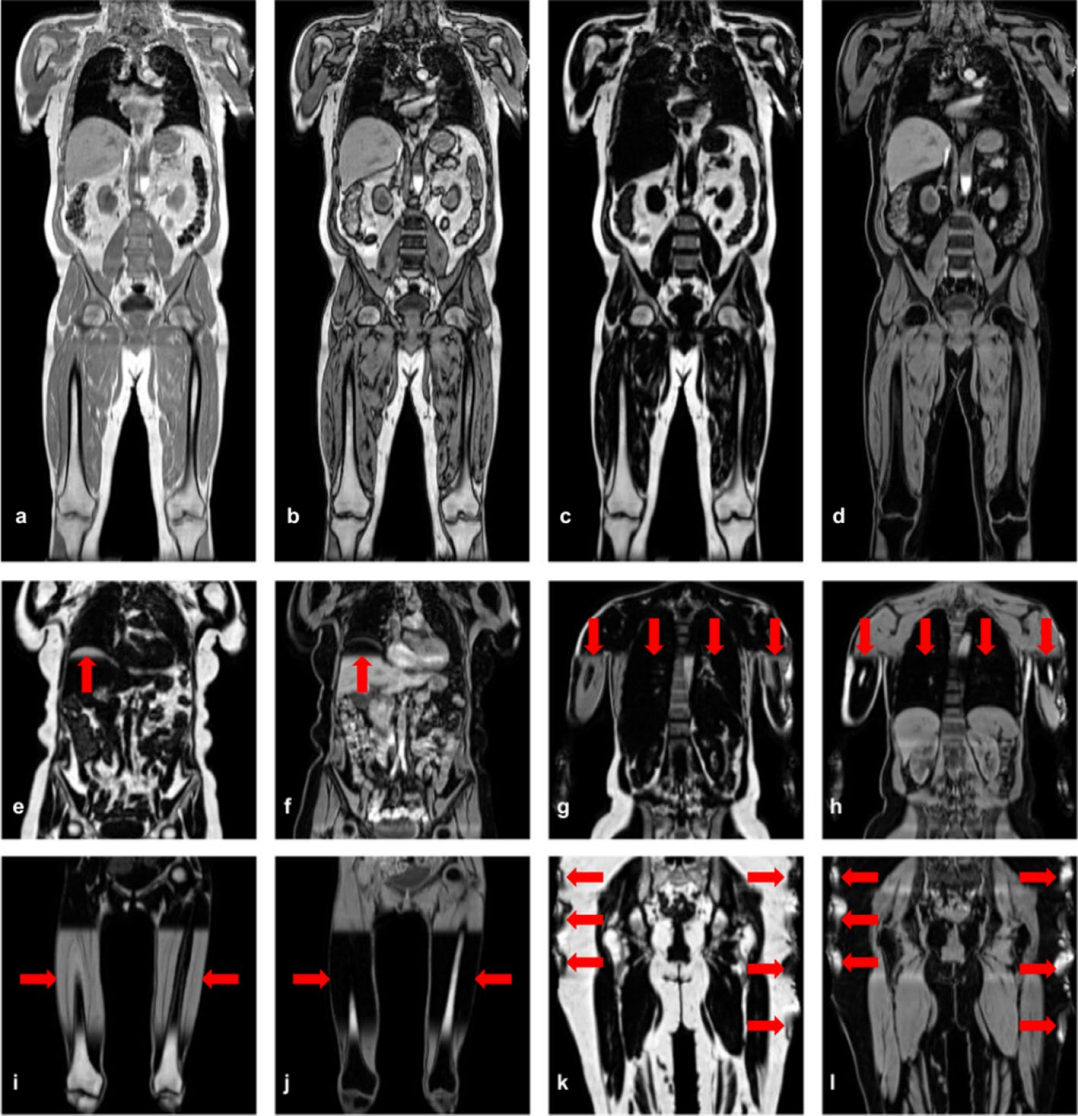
Dixon MRI data provided for representative UK Biobank participant: a in-phase, b opposed-phase, c fat and d water signals. Fat and water series with a partial swap e, f at the top of the liver, g, h a swap in a portion of the left arm and torso, i, j a swap in both legs and k, l localised swaps due to field inhomogeneities at the boundary of the field of view. Red arrows indicate fat-water swaps
